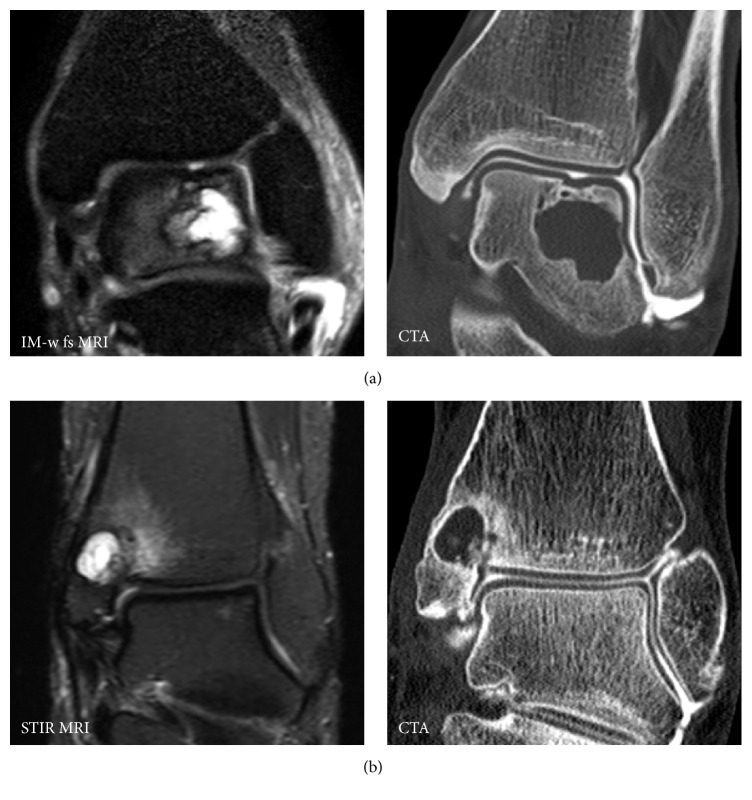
CTA for detection of full thickness cartilage lesions at the ankle in the presence of subchondral cysts. On MRI presence of full thickness cartilage defects remain unclear. In case (a) CTA demonstrates incongruence of the cartilage surface but no full thickness defect. In case (b) CTA demonstrated a fissural full thickness cartilage defect, allowing communication between intra-articular synovial fluid and subchondral cyst.
